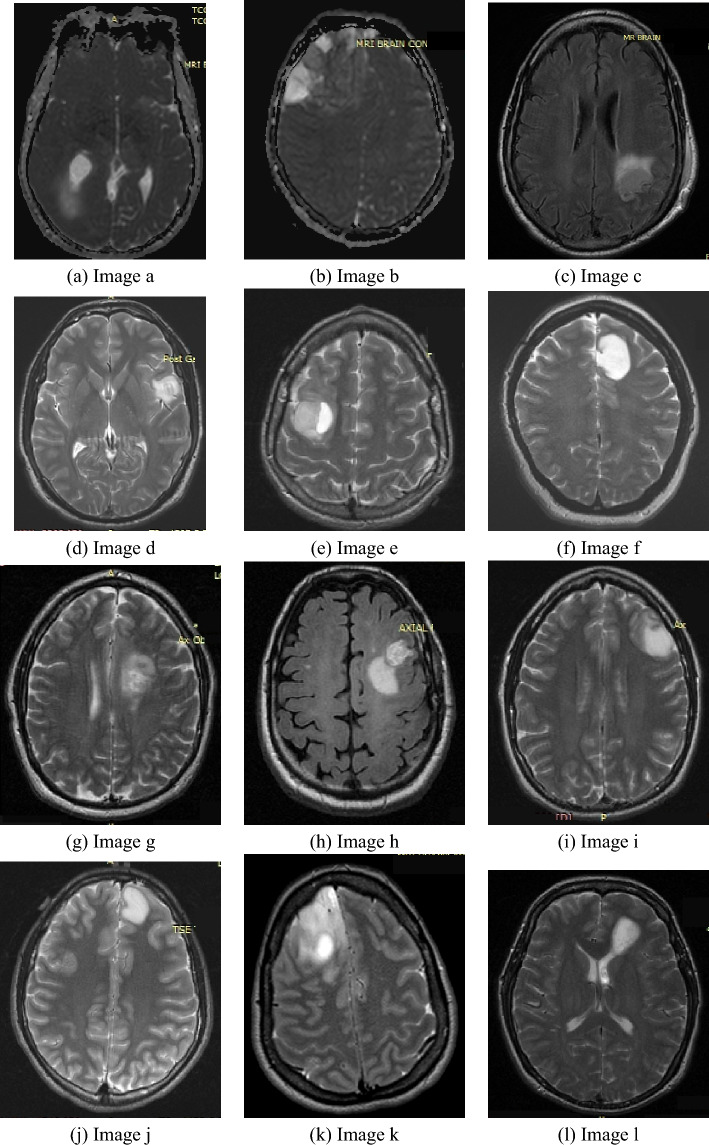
MRI Astrocytoma host images from the TCIA dataset used for watermark embedding
